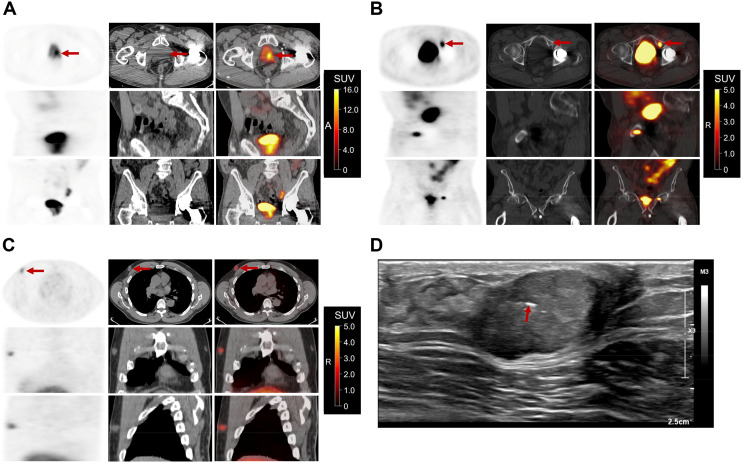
PET, CT, and fused PET/CT images showing [^18^F]DCFPyL uptake in vesicourethral anastomosis extending along bladder wall and neck (A), left superior pubic ramus metastasis (B), and right retroareolar mass (C). (D) Ultrasound-guided biopsy with microclip placement.
